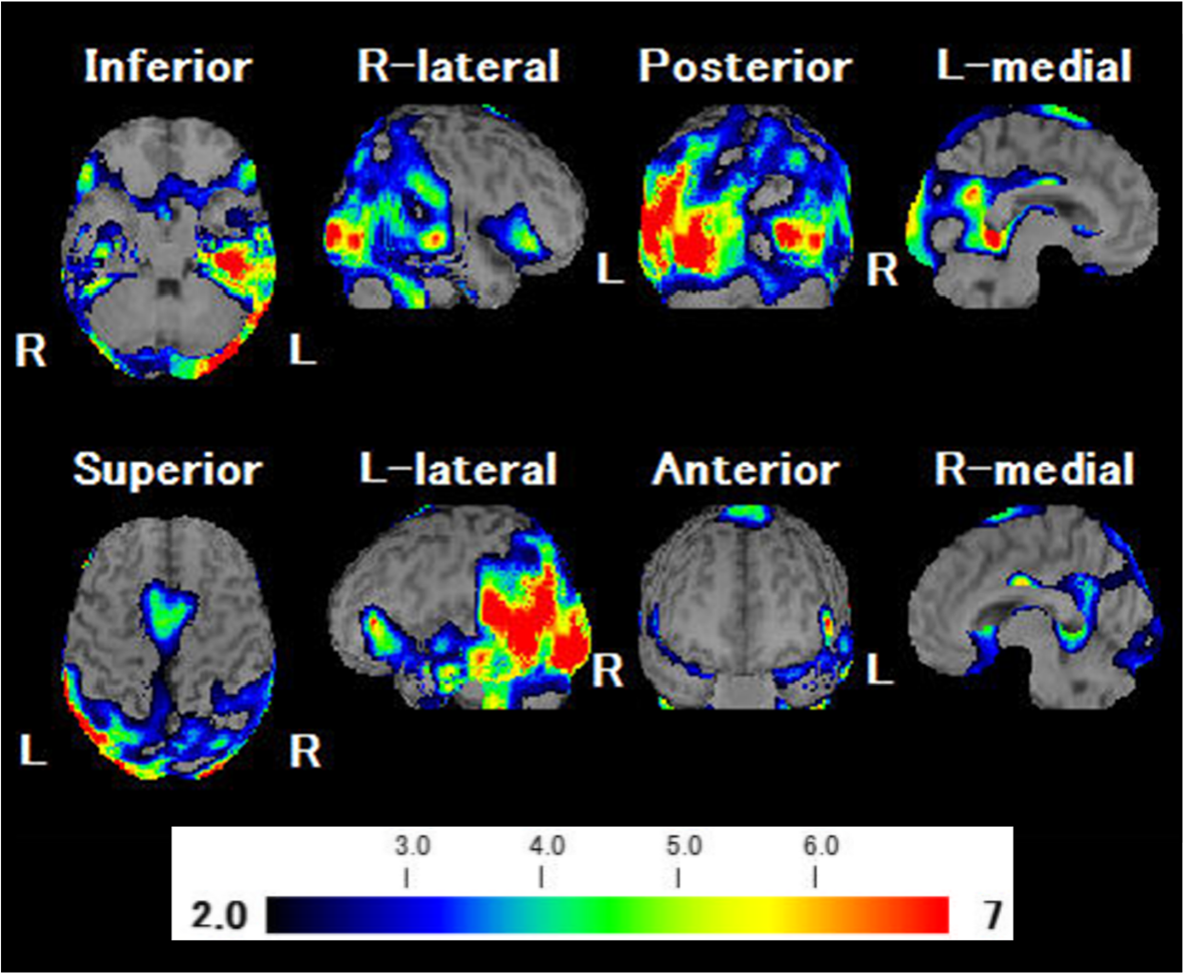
Brain single-photon emission computed tomography analysis with an easy Z score imaging system. *N*-iso-propyl-*p*-[^123^I] iodoamphetamine (IMP) single-photon emission computed tomography (SPECT) analysed with an easy Z score imaging system (eZIS) showing predominantly left-sided bilateral hypoperfusion of both the temporoparietal lobe and the posterior cingulate gyrus. L, left; R, right
